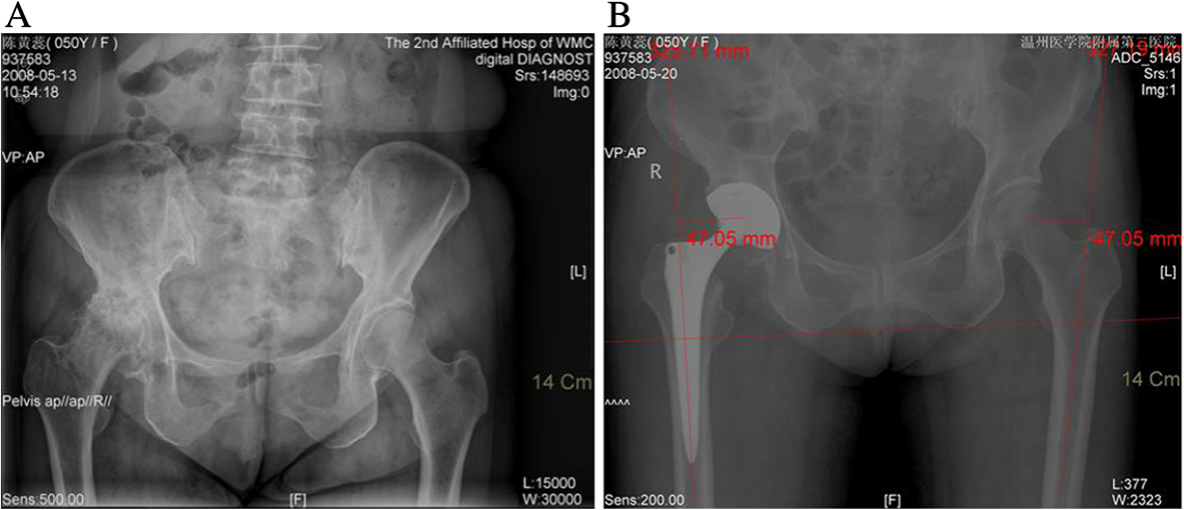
Radiographs of pelvis of a 50-year-old female. Presented with severe pain and limited range of motion in her right hip, secondary to osteoarthritis. (A) Preoperative limb-length inequality was 14.6 mm. (B) THA was performed using the Length-offset Lever (Figure 1). Postoperative limb-length inequality was 0 mm. The patient had excellent pain relief and functional results following the THA and no complications.
